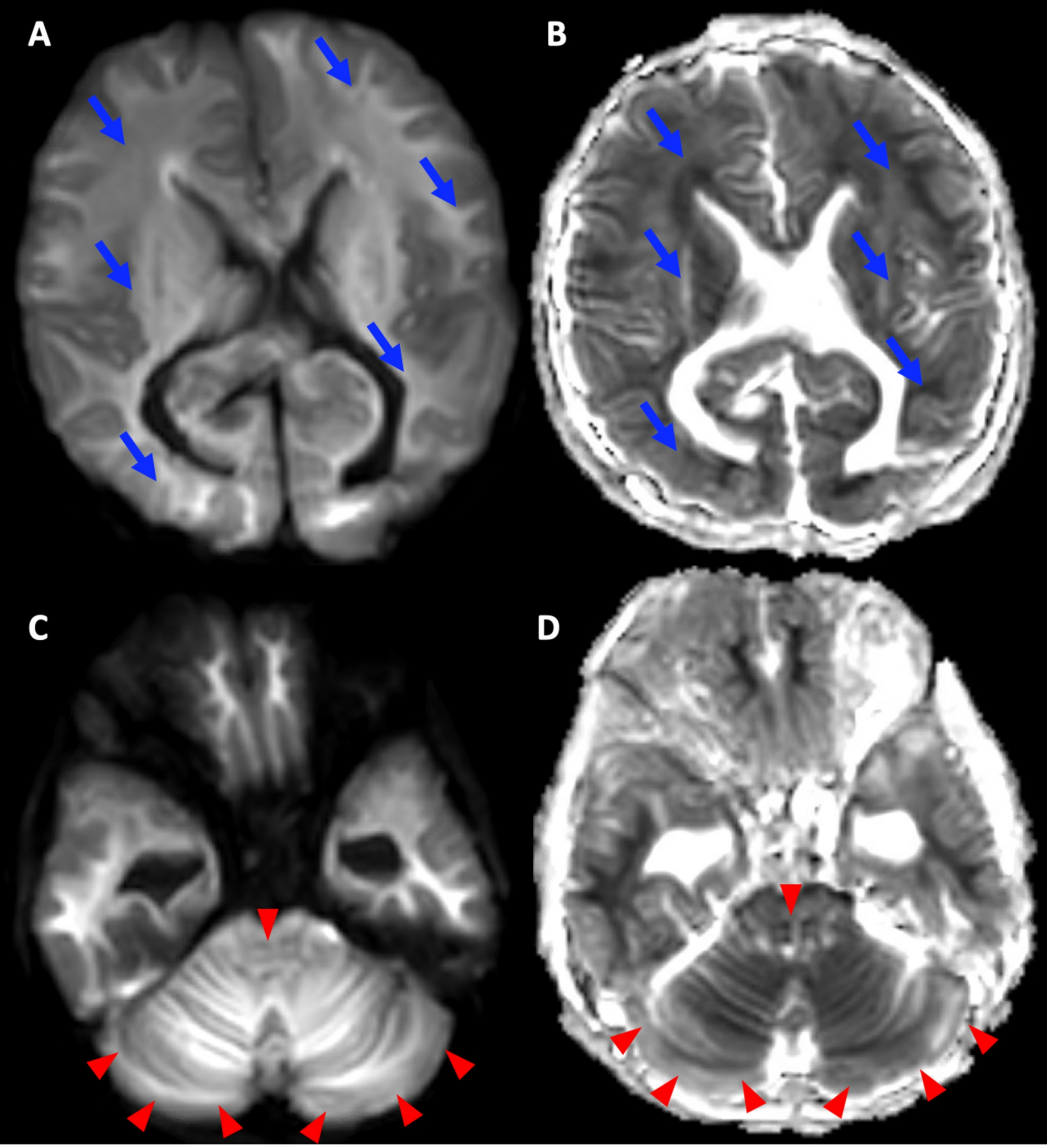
Follow-Up MRI Obtained One Month After Initial Imaging Image 3A, 3B: Isotropic diffusion-weighted image and apparent diffusion coefficient (ADC) maps demonstrated new, widespread diffusion restriction throughout the cerebral white matter (blue arrows). Images 3C, 3D: Isotropic diffusion-weighted image and ADC map demonstrated demonstrate new, widespread diffusion restriction in the cerebellum (red arrowheads).
